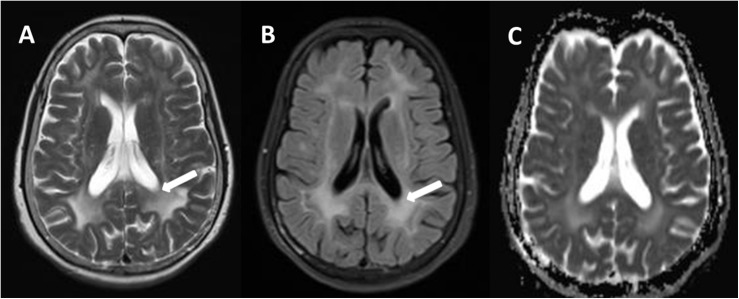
Comparison of a T2W (A) and fluid-attenuated inversion-recovery (FLAIR) (B), and apparent diffusion coefficient (ADC) (C) in transverse (axial) cross section through hemispheres at lateral ventricles. There are relative preservation of the periventricular region (A, arrows) and sparing of the subcortical U fibers on T2W. Compared to T2W, intensity of periventricular rims is suppressed in the most severely affected areas (B, arrows). The hyperintense lesion areas in ADC were smaller on T2W (C).
